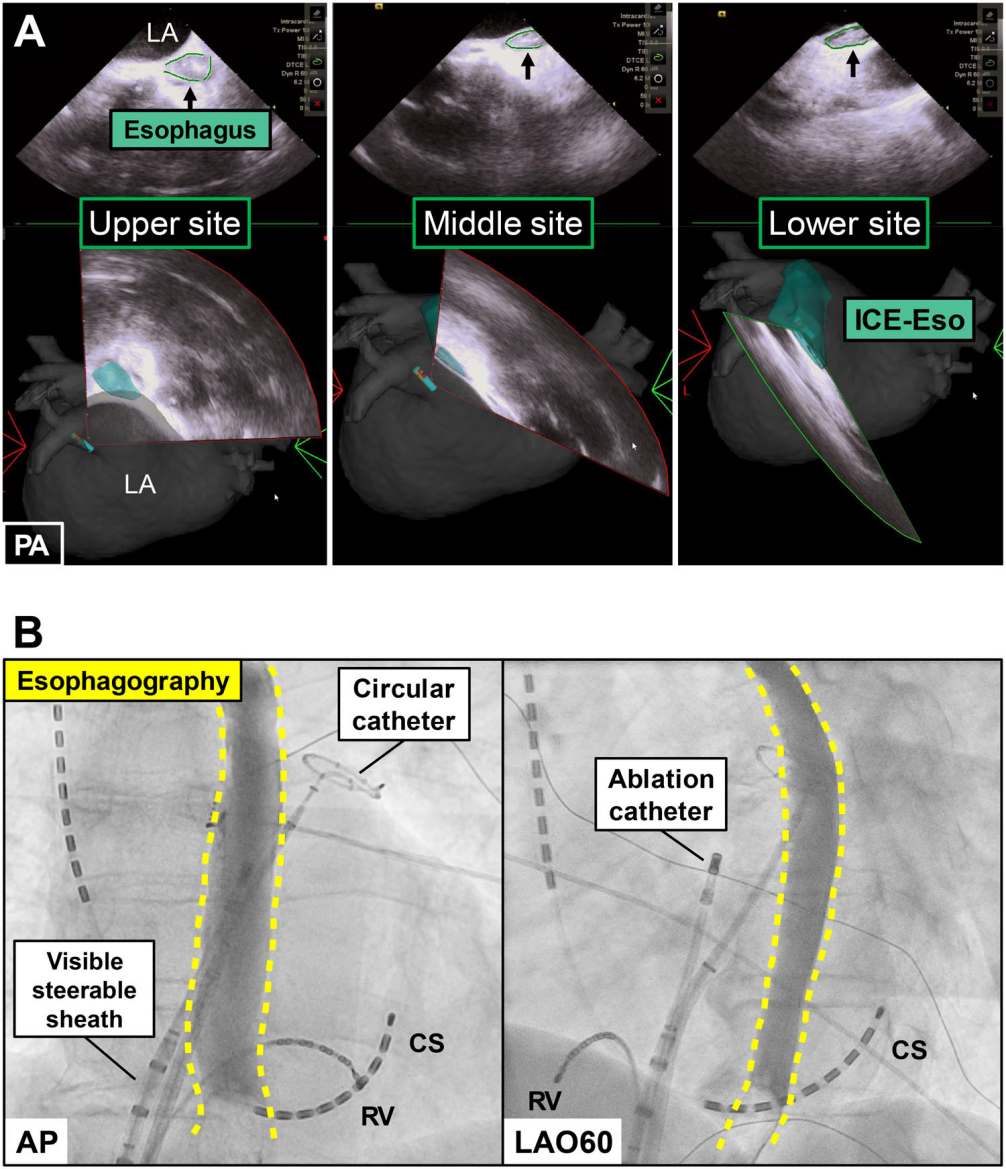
Esophageal Imaging using Intracardiac Echocardiography (ICE‐Eso) and Contrast Esophagography. Panel A: The esophagus using intracardiac echocardiography is shown on three sections in the *green area* (*Left panel*: Upper site, *Middle panel*: Middle site, *Right panel*: Lower site). Panel B: Contrast esophagography using gastrografin shows real‐time esophageal location (*yellow dotted line*) in the anterior‐posterior view (*left panel*) and the left anterior oblique view (*right panel*).
